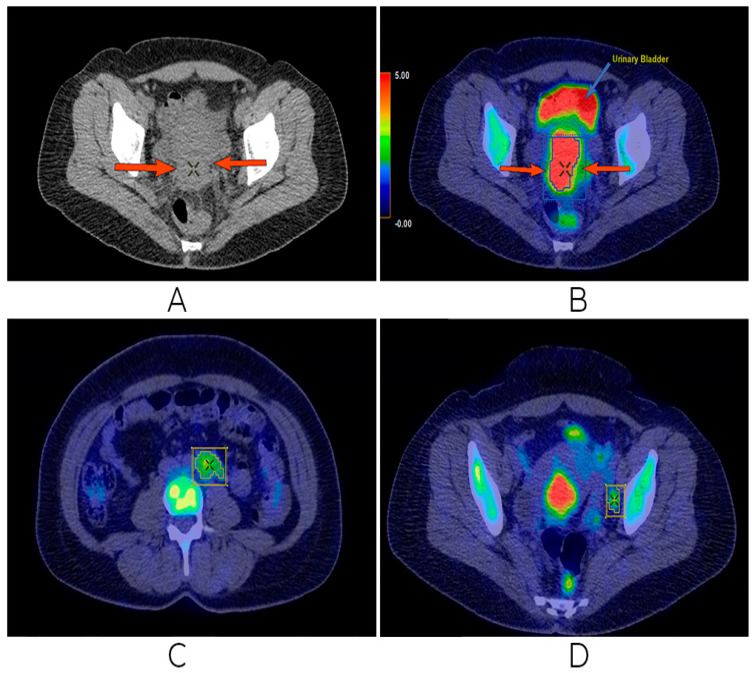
First FDG PET/CT examination. High FDG uptake in uterine cervical lesion (A,B), left para-aortic retroperitoneal (C), and left pelvic (external iliac) (D) adenopathies, both with FDG uptake.
